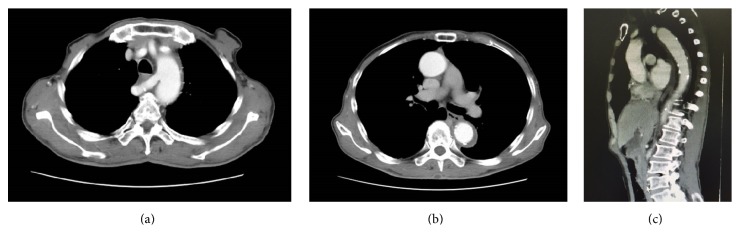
Contrast-enhanced CT images at time of onset. (a, b) Horizontal cross section. The origin of the right subclavian artery is identified as the fourth branch of the aortic arch and runs posteriorly to the right of the esophagus. No dissection of the right subclavian artery was present. Thrombosis of the false lumen from the origin to periphery of the right subclavian artery was observed. (c) Sagittal section. False lumen was observed. Total false lumen thrombosis was observed, and no signs of ULP were noted.
